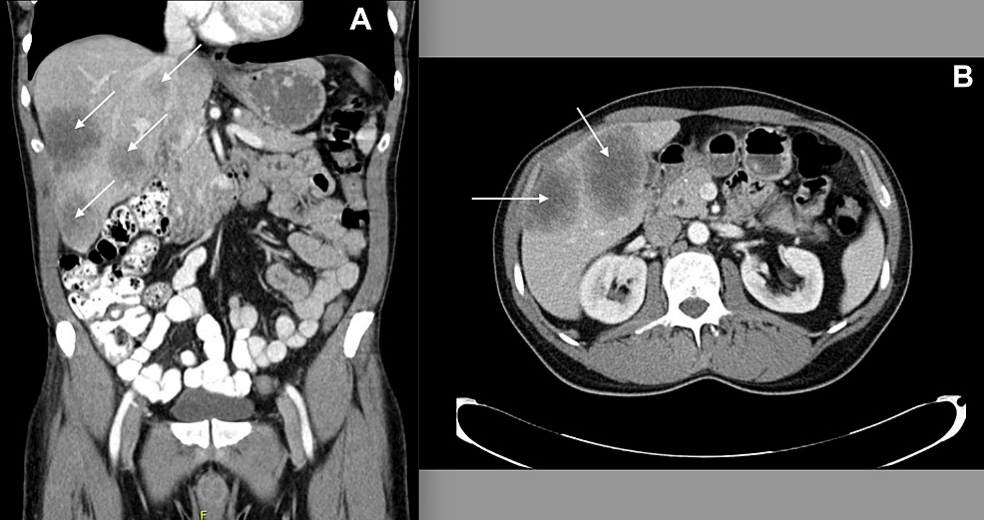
CT imaging of bi-lobar hepatic metastases (white arrows). A: coronal view; B: axial view. CT: computed tomography
